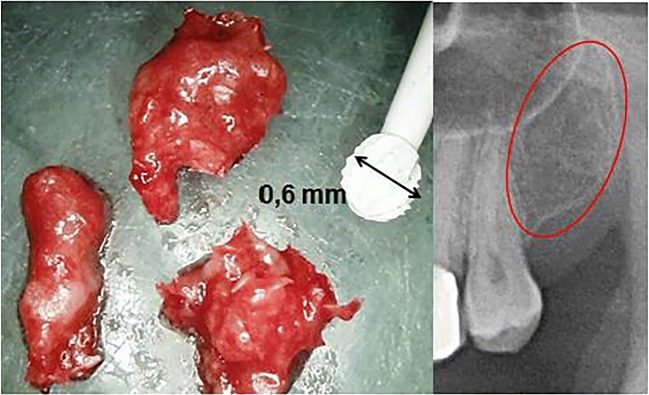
Left panel: Fatty and osteolytic spongy bone samples of medullary jawbone. Right panel: The corresponding radiograph of an apparently unaltered area of the upper left jawbone (28/29)
